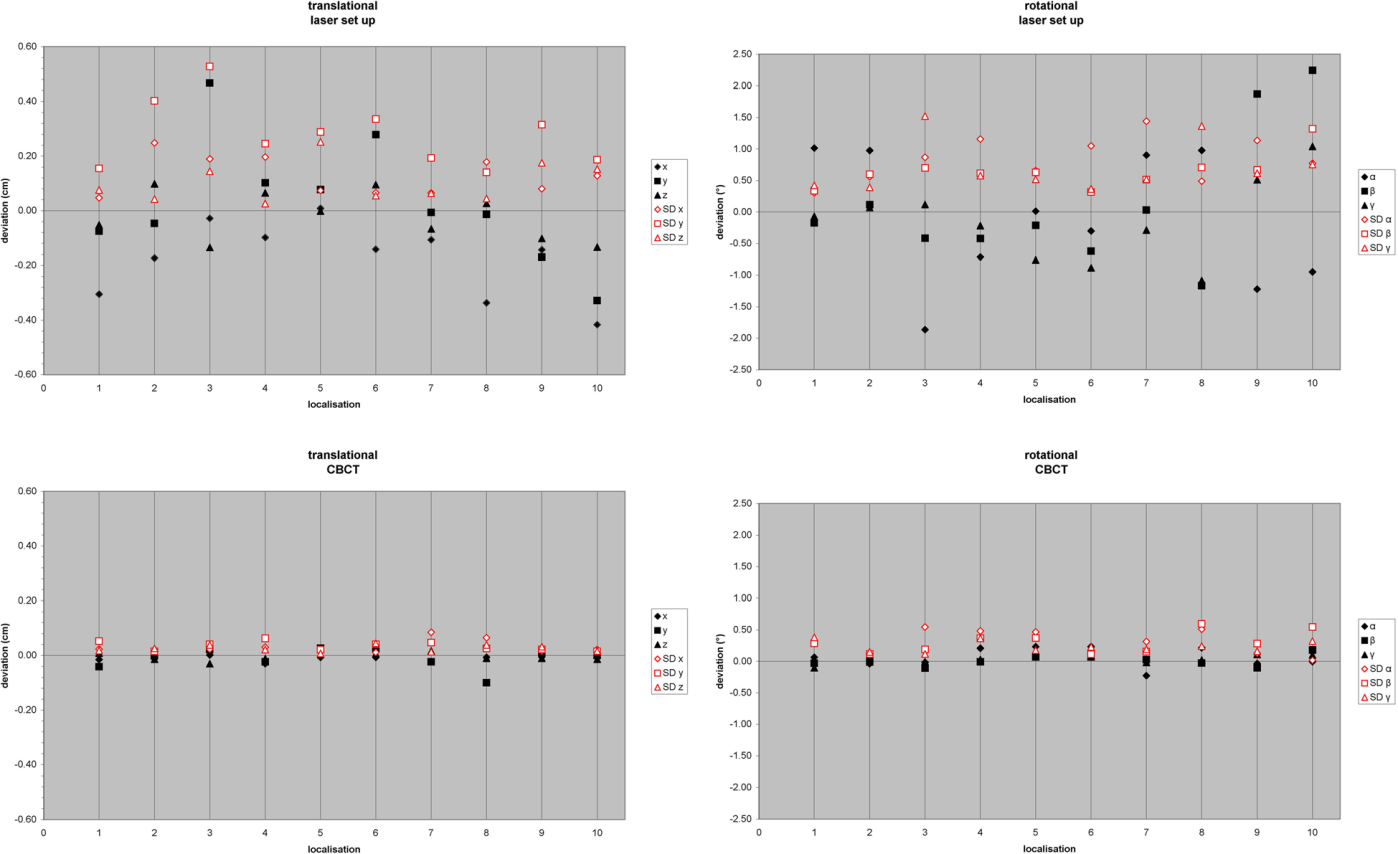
Average translational (left) and rotational (right) setup error and SD for each lesion for laser based (top) and CBCT based (bottom) patient setup.
